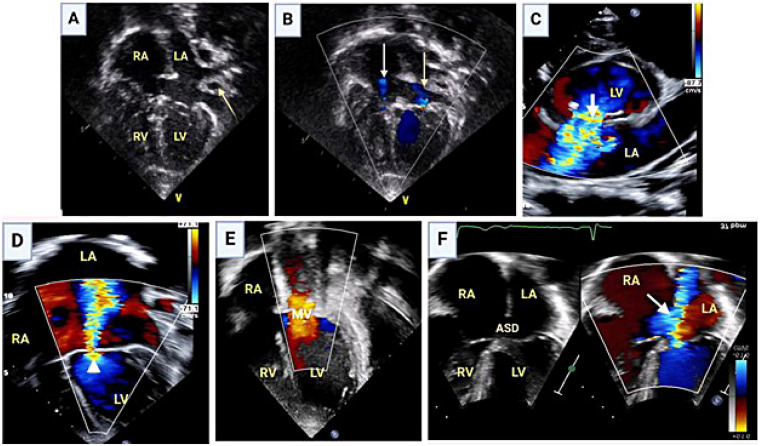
Echocardiography of the patients. (A) Echocardiography with the apical 4-chamber view of the first patient was done during the last follow-up, showing a dilated coronary sinus due to persistent left SVC (yellow arrow). (B) An apical 4-chamber view with color flow Doppler of the first patient was performed at the last follow-up and showed mild TR (white arrow) and mild MR (yellow arrow). (C) Preoperative modified parasternal short-axis view of the second patient showing a large cleft in the anterior mitral leaflet with severe MR (white arrow). (D) Preoperative apical 4-chamber view of the second patient with color flow Doppler showing MR jet passing through the body of the anterior mitral leaflet (arrowhead). (E) An apical 4-chamber view of the second patient after the mechanical mitral valve prosthesis, showing laminar blood flow across the mitral inflow. (F) Preoperative apical four-chamber view in 2D (left) and color flow Doppler (right) of the father showing ASD primum with a cleft in the anterior mitral leaflet causing mild to moderate MR (white arrow). ASD, atrial septal defect; LA, left atrium; LV, left ventricle; MV, mitral valve; MR, mitral regurgitation; RA, right atrium; RV, right ventricle; SVC, superior vena cava; TR, tricuspid regurgitation.
